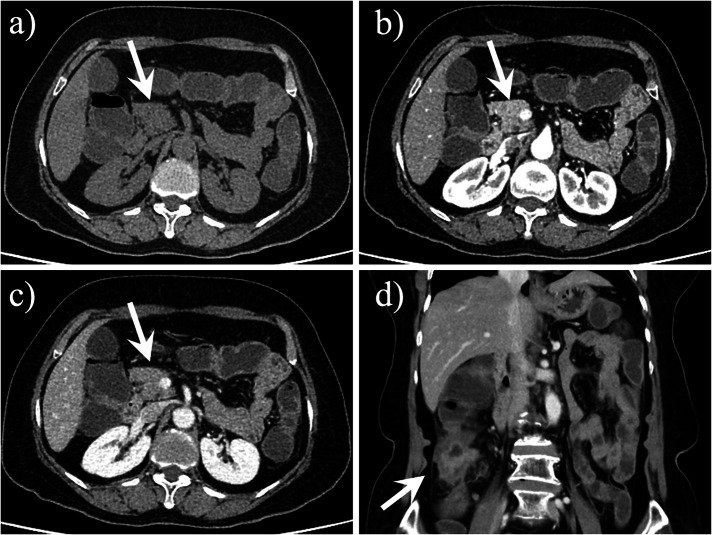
A 76-year-old woman with dorsal pancreatic agenesis coexisted with colon cancer. Axial unenhanced computed tomography (CT) image (a) and contrast-enhanced CT images (b, c) showed the pancreatic head (arrow) and the absence of the pancreatic body-tail portion. Coronal contrast-enhanced CT image (d) showed marked irregular wall thickening in the proximal ascending colon‌ (arrow), with postoperative pathological examination confirming the diagnosis as colorectal adenocarcinoma‌
